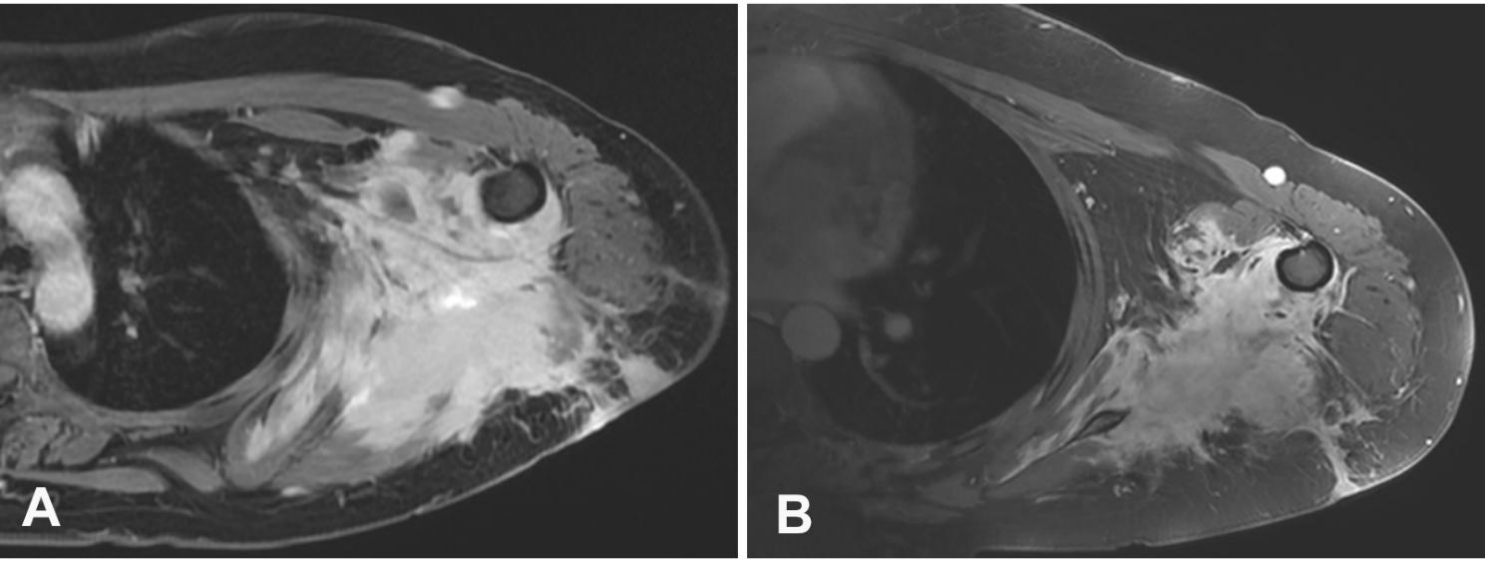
(A) Solid 147-mm mass in the left upper arm (MRI scan) before the treatment. (B) After one month of TKI treatment, the max size of mass was reduced to 108 mm (MRI scan)
